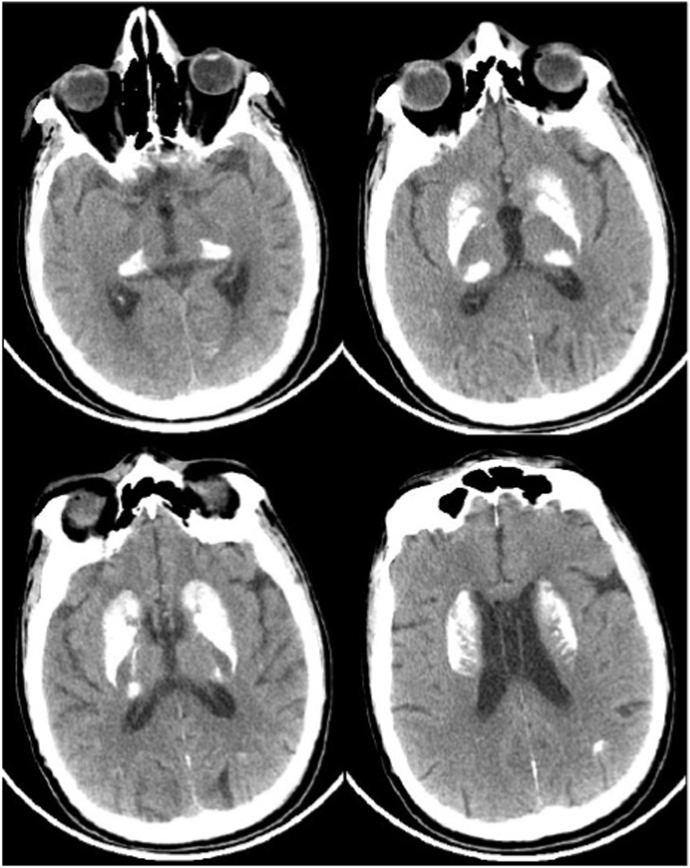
Brain CT; showed extensive symmetric bilateral basal ganglia calcifications.
